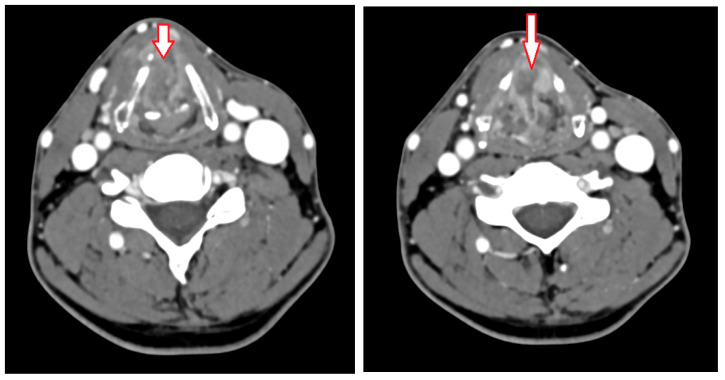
CT-scan images before the treatment showing the tumor (arrow).
